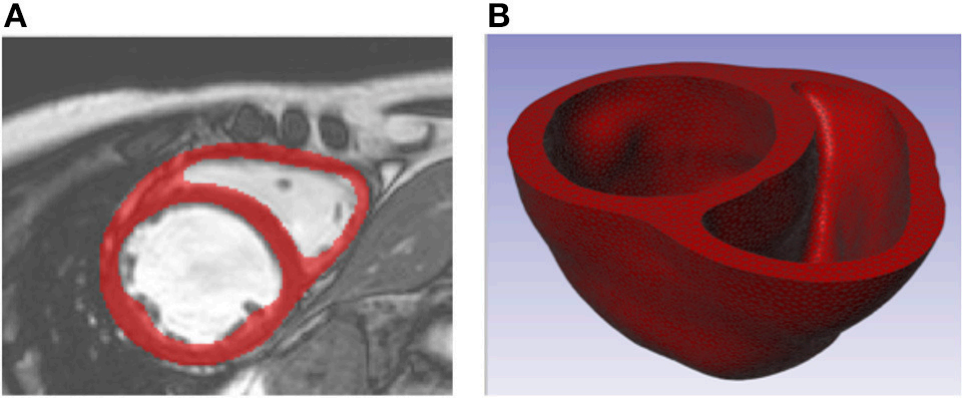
(A) Short axis MR image of patient with dilated chronic heart failure overlaid with the segmentation of the myocardium. (B) Truncated biventricular geometry extracted from segmentation and meshed using tetrahedral elements.
